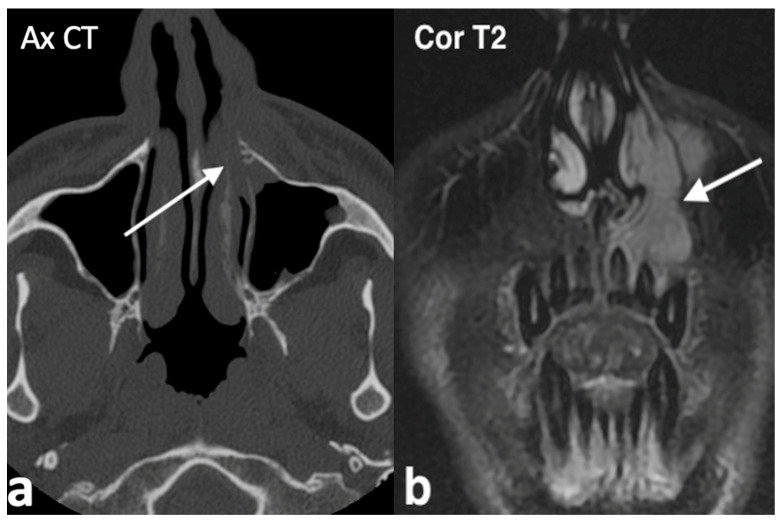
Non-Hodgkin lymphoma in a patient with monolateral left nasal obstruction. Rhinoscopy highlights a mass with a regular smooth surface responsible for raising mucosal lining of nasal floor. The axial CT image (a) shows a lobulated thickening of mucosal lining of the left lateral nasal wall (arrow), also extending to the maxillary sinus; note that lymphoid tissue permeates the adjacent cortical bone of the medial wall of the maxillary sinus. On the MRI coronal T2 fat saturation image (b), the lesion (arrow) shows high intensity, and extends into the lateral aspect of the left inferior turbinate, invading the left half of maxillary bone.
